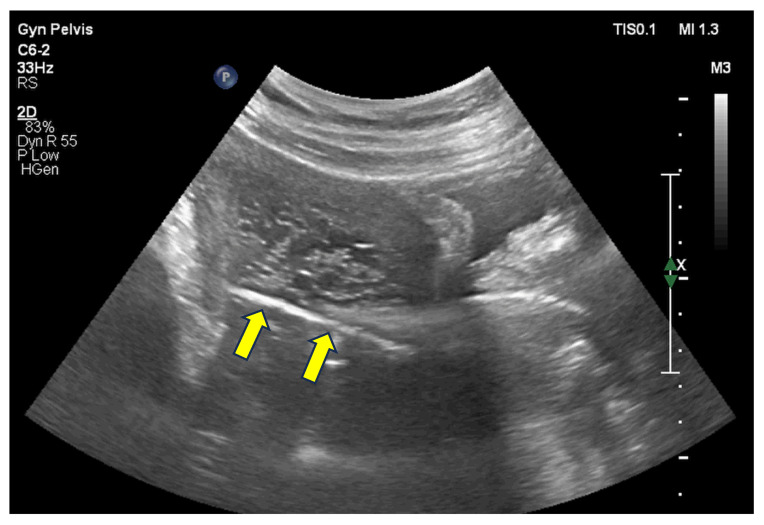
Pelvic ultrasound used to guide uterine evacuation for the treatment of molar pregnancy. Yellow arrows indicate the uterine aspiration cannula suctioning molar tissue. Ultrasound guidance during evacuation reduces the risk of uterine perforation and minimizes residual trophoblastic tissue.
